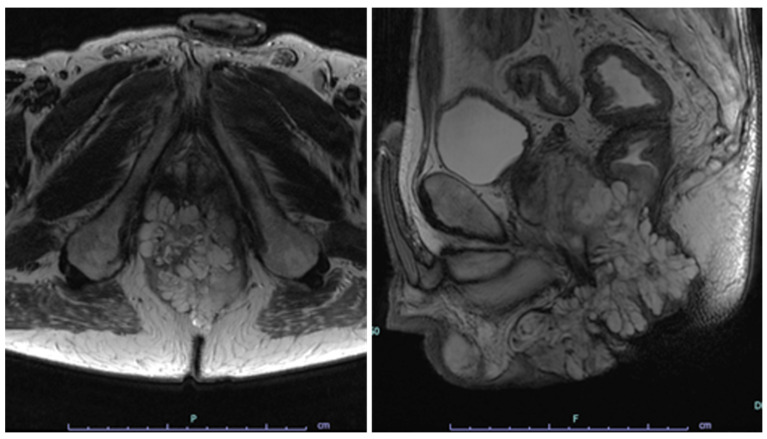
MRI T2-weighted images of a case of anorectal cancer, axial (left) and sagittal (right). A multilocular cystic lesion is seen in the rectum and anal canal.
